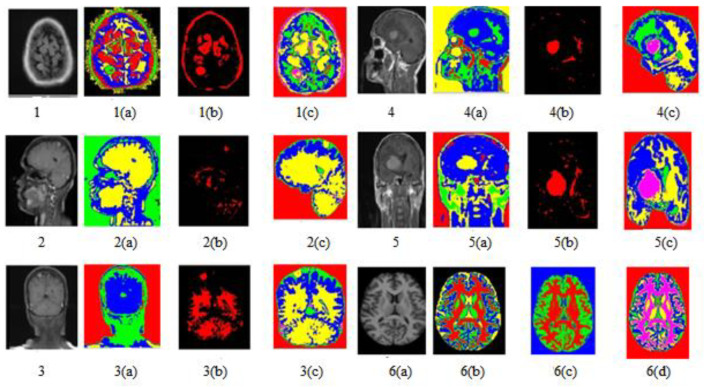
Image segmentation of T1, T2, and FLAIR image sequences.
